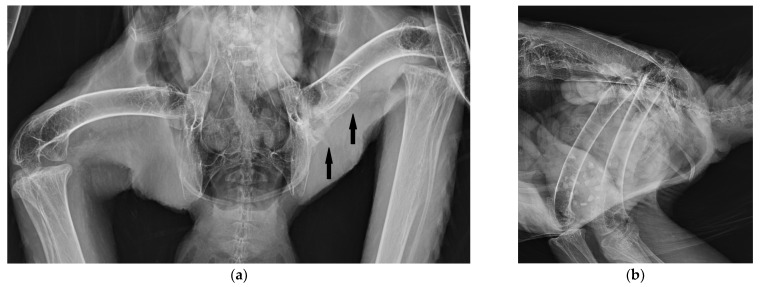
Ventro-dorsal (a) and latero-lateral (b) radiographs of a bald eagle (case 3) with heterotopic bone formations (arrows), with a smooth surface on almost the entire distance between pelvis and femur in the course of the adductors. The bone formations look like mature lamellar bone.
